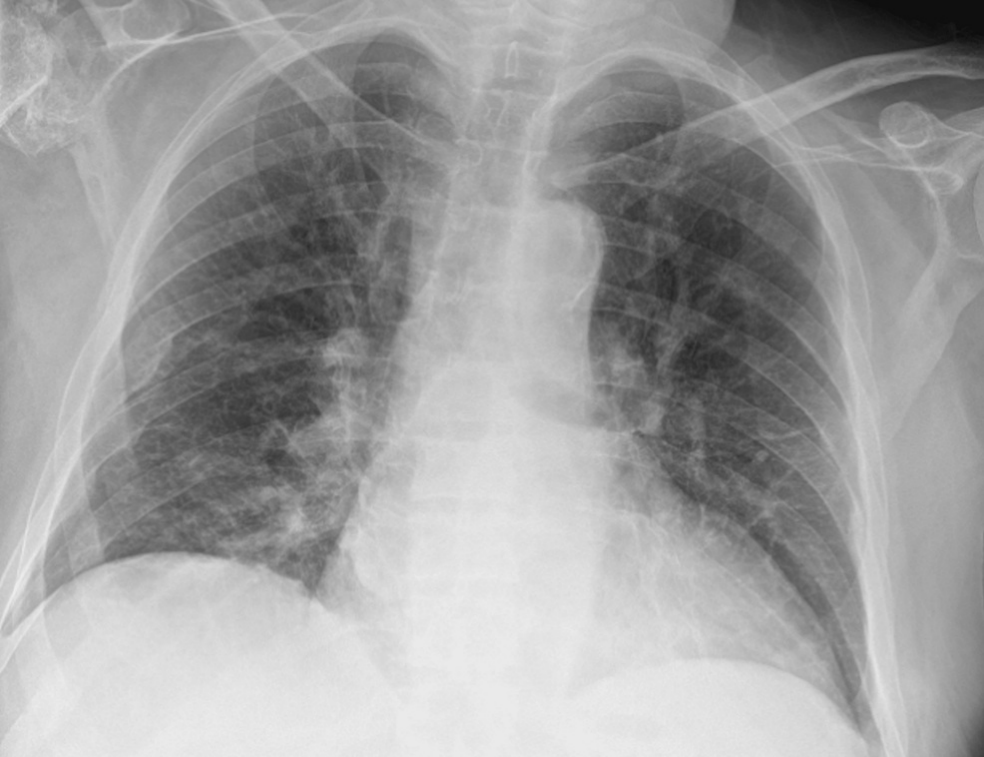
AP chest x-ray showing right middle zone consolidation, in keeping with pneumonia. AP: anteroposterior
